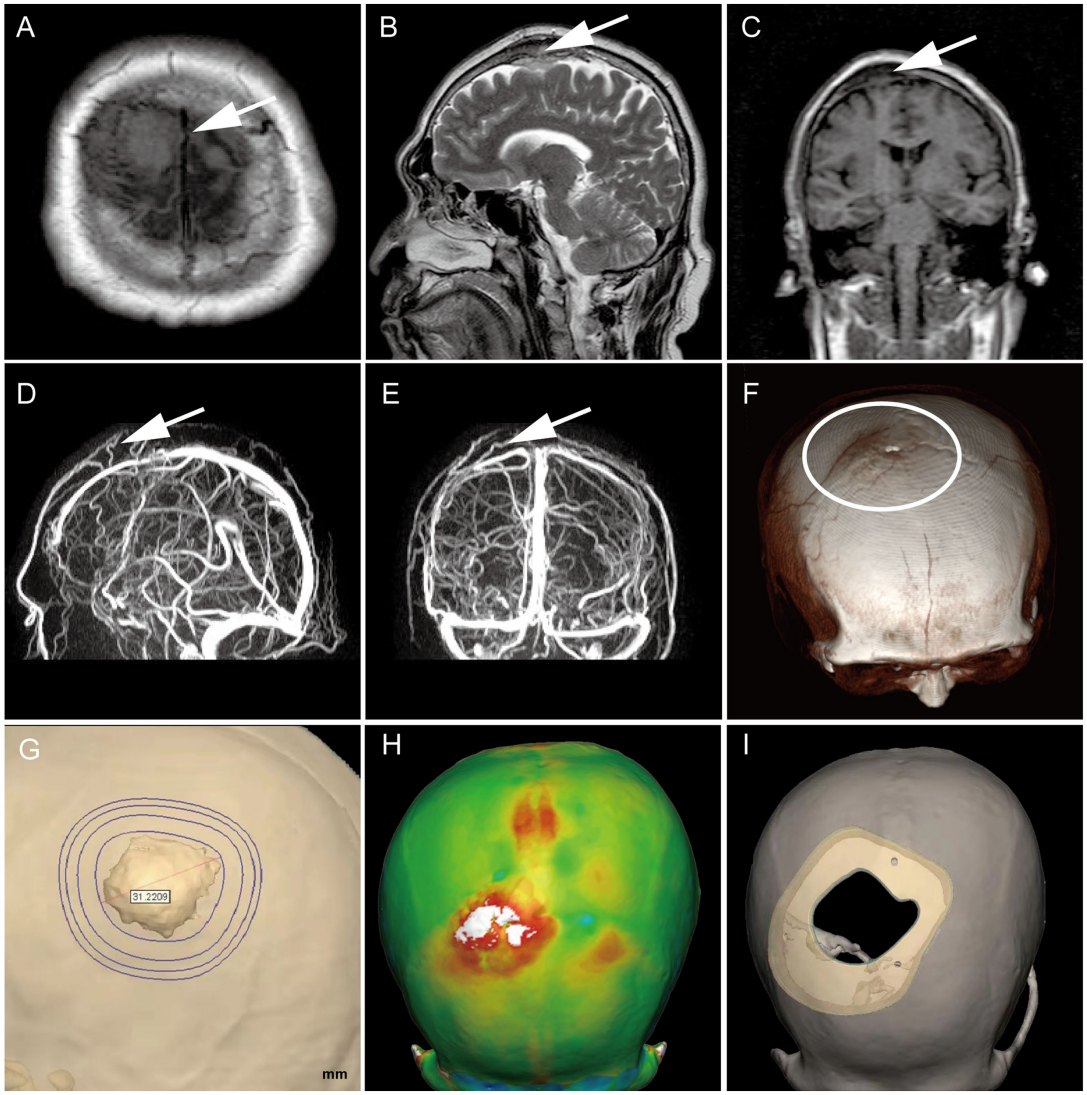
Preoperative MRI confirmed the tumor’s location (white arrow), with T1 low signal and T2 equal high signal, invading the skull and compressing brain tissue locally (A–C).MRV suggests that it is closely related to the branches of the sagittal sinus (D,E); Preoperative CT three-dimensional reconstruction showed a slight skull bulge at the lesion site (F); Determine the skull repair scheme by three-dimensional modeling (G–I).
